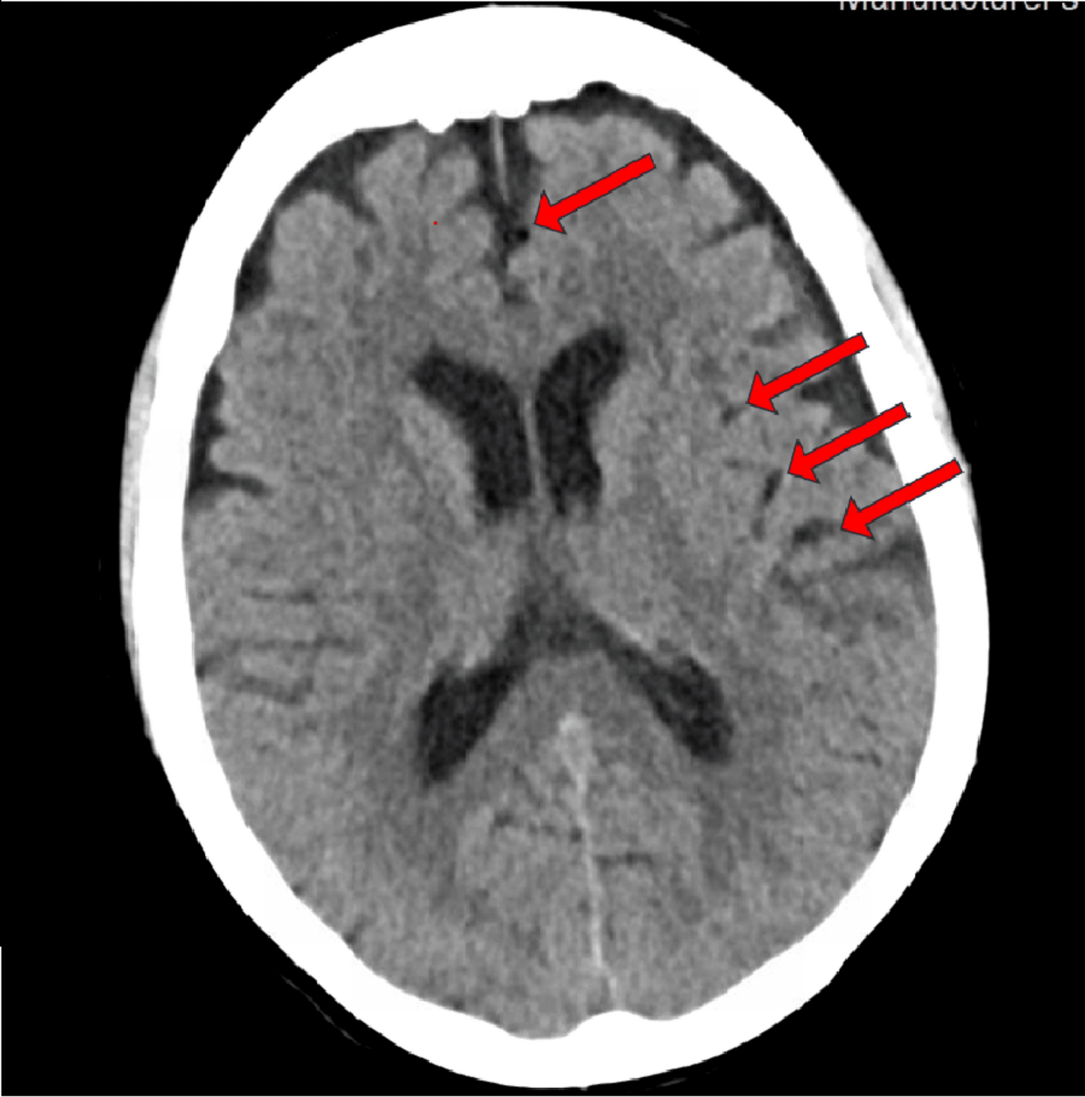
Computed tomography (CT) of the brain revealed air emboli present throughout the left cerebrum (indicated by the red arrows)
